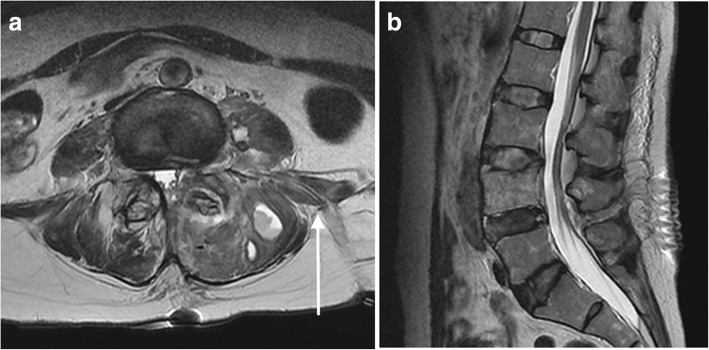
Magnetic resonance images of the lumbar vertebra at 1 week after surgery. a Axial and b sagittal T2-weighted magnetic resonance imaging showing the marked improvement of the psoas abscess. The *white arrow* shows the drainage tube from the psoas abscess
